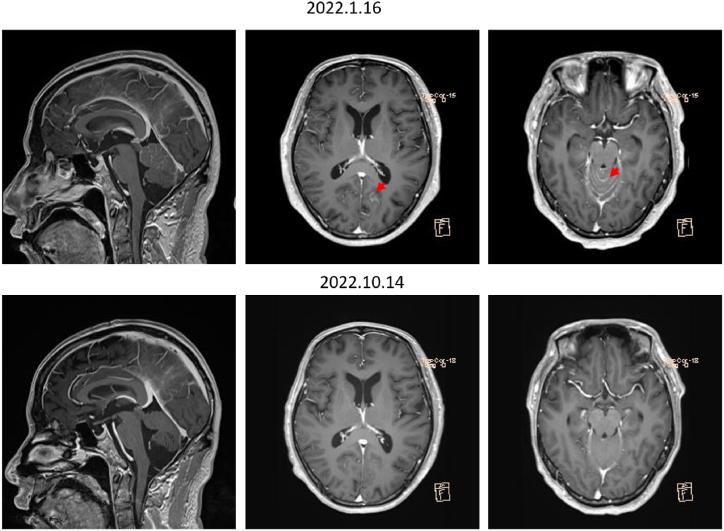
Contrast-enhanced MRI of the head with sagittal view (left panels) and axis view (middle and right panels) of patient one. The red arrow indicates meningeal dissemination. MRI, magnetic resonance imaging. (For interpretation of the references to colour in this figure legend, the reader is referred to the Web version of this article.)
